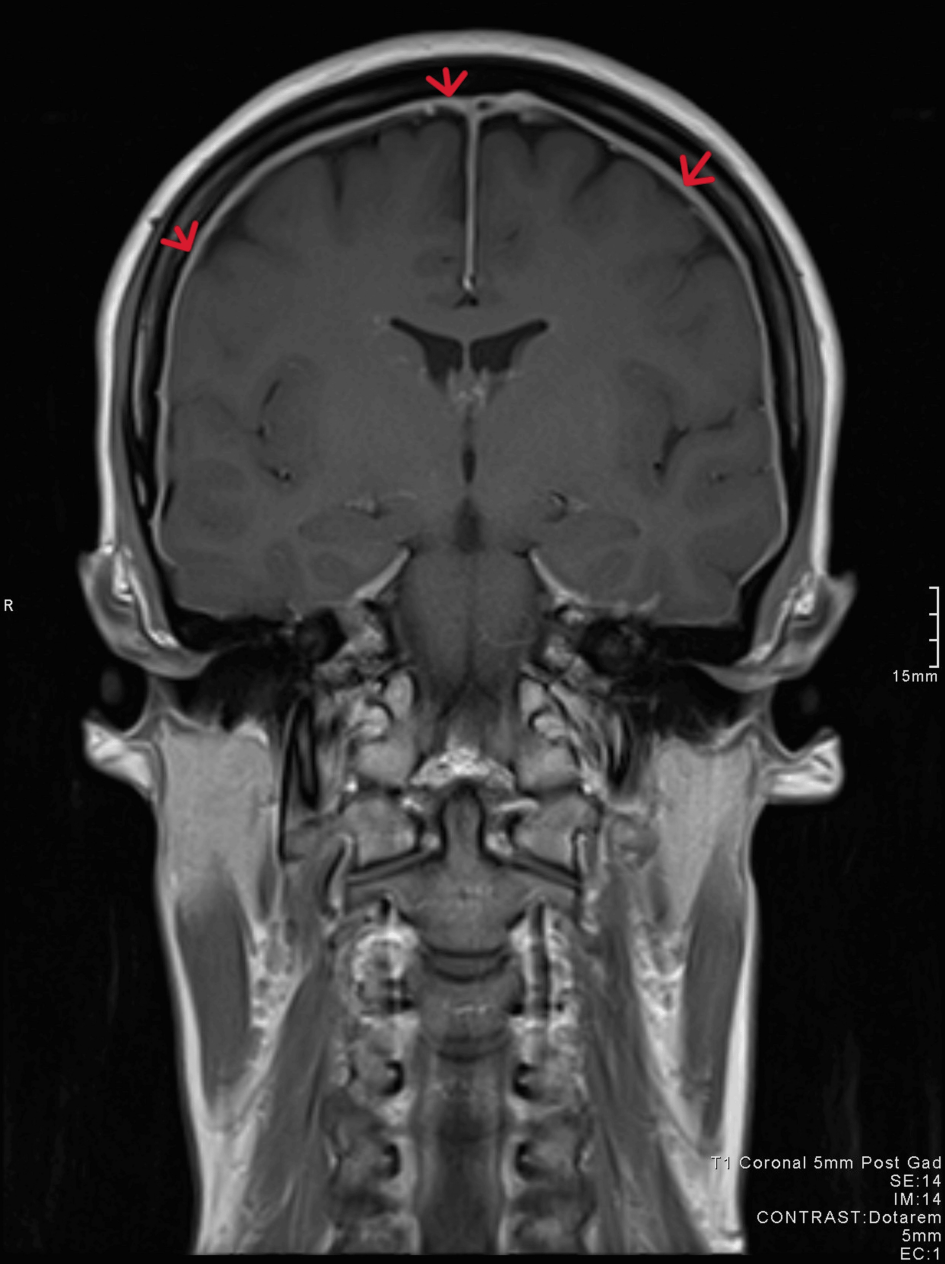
MRI coronal view showing pachymeningeal enhancement.
